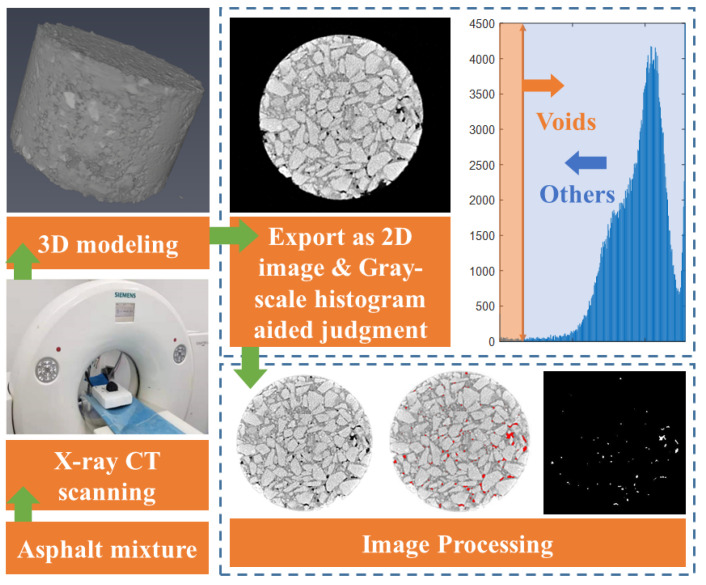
CT scanning and image processing.
